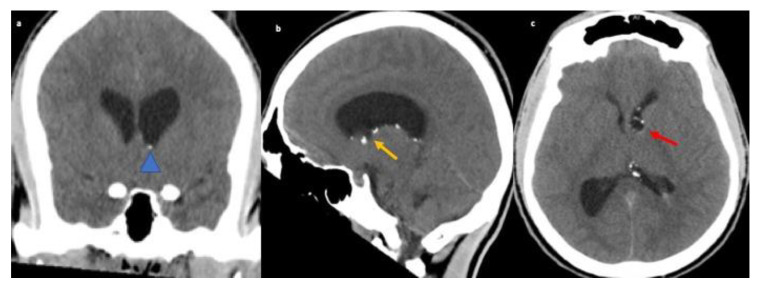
CT Head non-contrast coronal (a) reveals small punctuate foci of calcification along the left lateral ventricle (blue arrow head). Additional CT head sagittal and axial (b,c) reveals 9 mm × 6 mm fluid density lesion in the frontal horn of left lateral ventricle with peripheral calcification near foramen of Monro (yellow and red arrow) leading to obstructive supratentorial hydrocephalus. Additional small punctuate foci of calcification along the left lateral ventricle.
